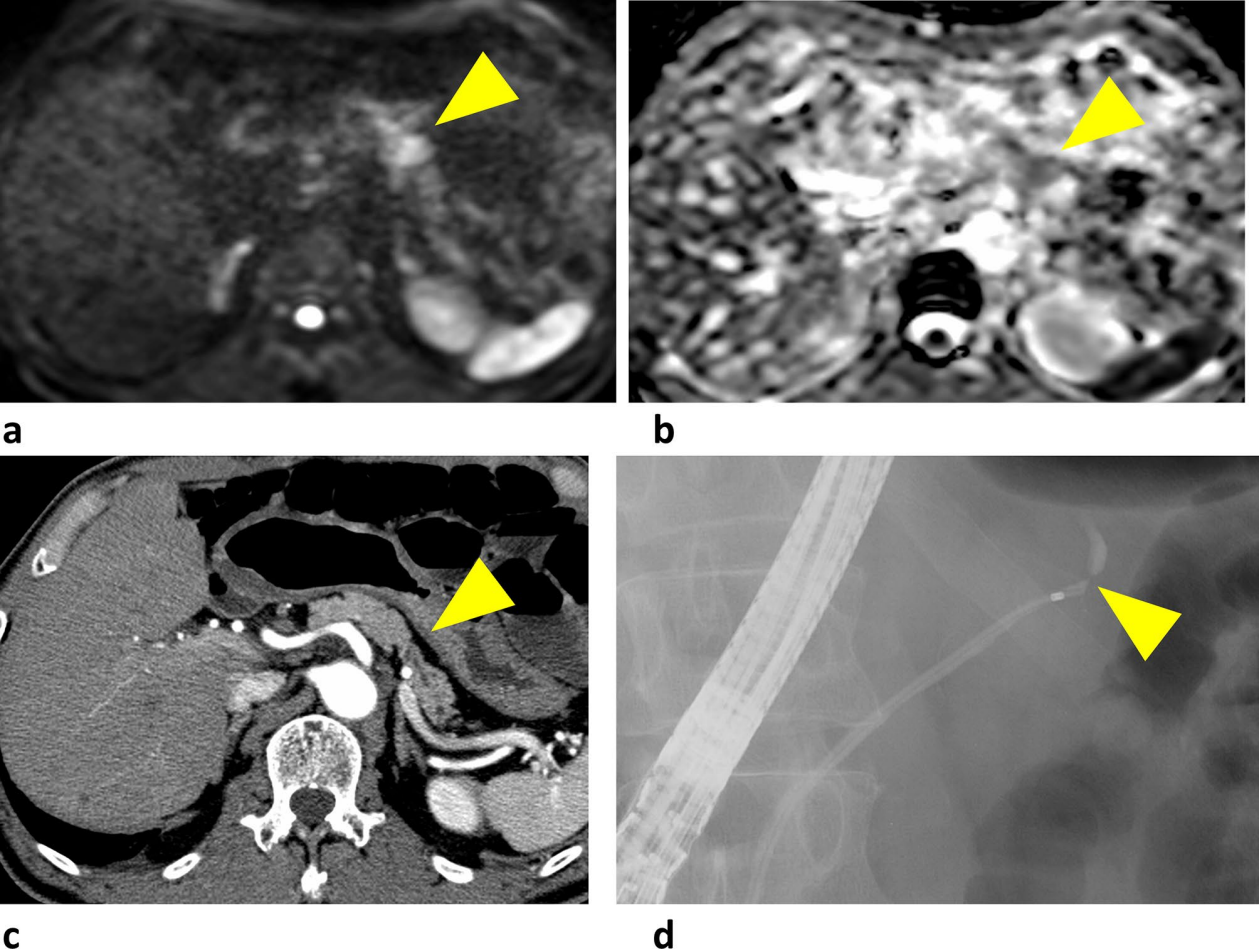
a High signal intensity around the stricture in the diffusion-weighted image (arrowhead). b apparent diffusion coefficient values by 2 readers were 1.65 and 1.62 (× 10^−3^mm^2^/s), respectively. c Focal pancreatic parenchyma atrophy and main pancreatic duct stricture without a visible mass on computed tomography (arrowhead). d pancreatography shows a high-grade pancreatic duct stricture in the pancreatic tail
